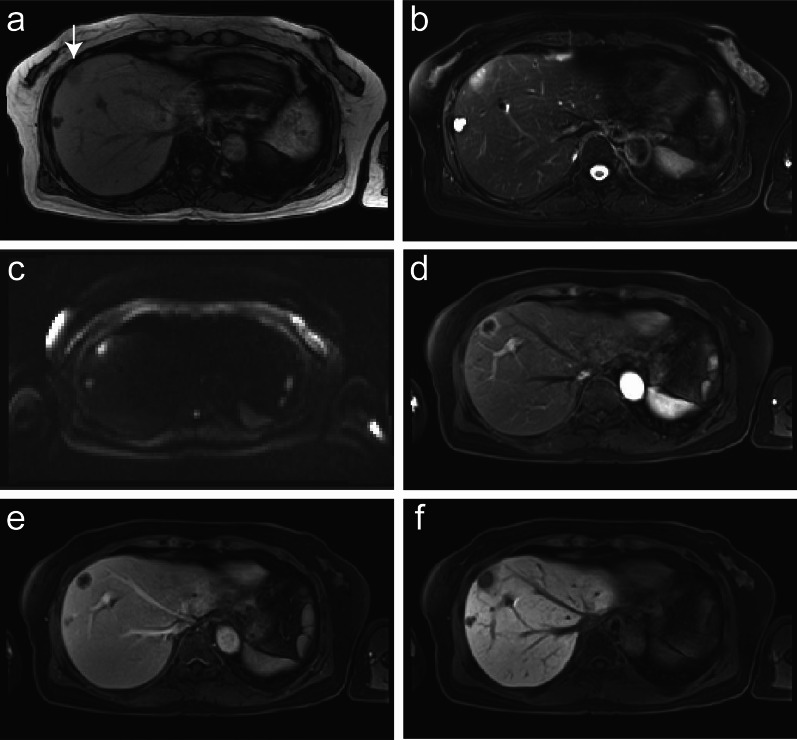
Magnetic resonance imaging (MRI) and gadolinium-ethoxybenzyl-diethylene-triaminepentaacetic acid (Gd-EOB-DTPA) MRI. MRI shows low intensity on T1-weighted (a) and high intensity on T2-weighted (b) and diffusion-weighted (DWI) images (c). Enhanced MRI shows marginal enhancement in the arterial phase (d) and venous phase (e) and hypointensity in the hepatocellular phase (f)
